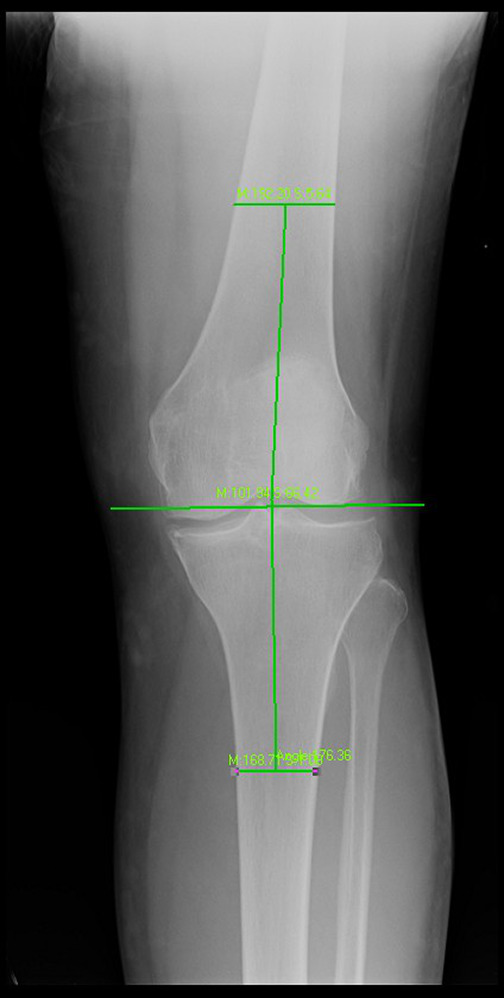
Affected knee joint orthotopic radiography at non‐weight‐bearing position (supine position).
